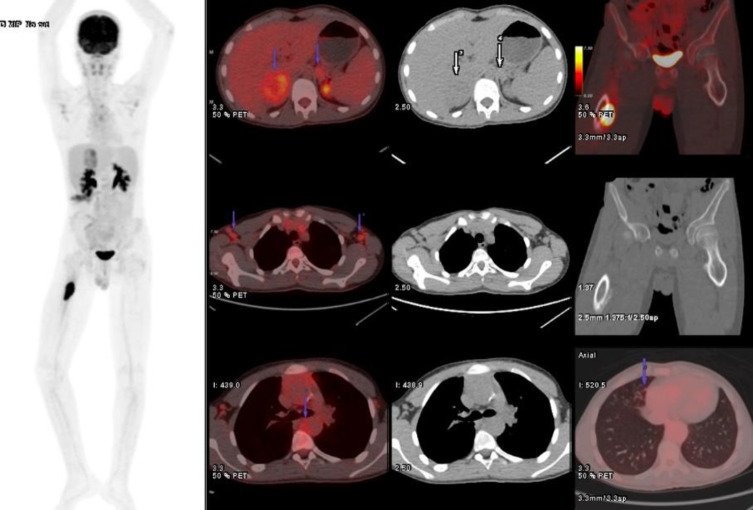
17 y/o patient with history of HIES underwent PET/CT scan due to right femoral pain. The study is suggestive of FDG avid right adrenal mass (measuring 51×55mm, SUV_max_= 5.4), mildly FDG avid left adrenal nodule (measuring 18×12mm, SUV_max_=2.7) and few pulmonary nodules with mild FDG uptake, largest one located in RML (measuring 6mm, SUV_max_=1.4), hypermetabolic lytic sclerotic right femoral osseous lesion along with periosteal reaction (SUV_max_=4.6) and multiple hypermetabolic bilateral axillary lymph nodes, some with thick cortex (measuring up to 12×12mm, SUV_max _up to 2.9)
